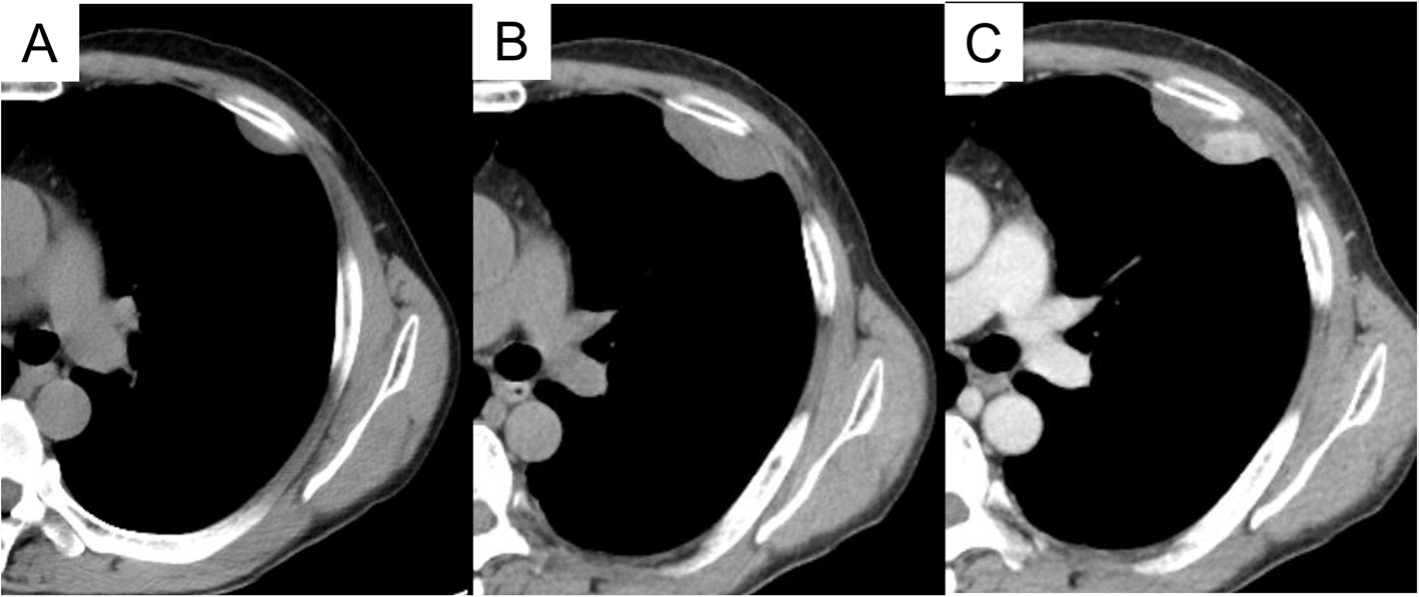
Chest computed tomography (CT) showing the tumor on the left chest wall in the thoracic cavity. On CT, the tumor size was 27 mm at the annual medical checkup (A) and had increased to 50 mm six months after the checkup (B). Contrast-enhanced CT showed a contrast-enhancing area within the tumor at the same time (C)
